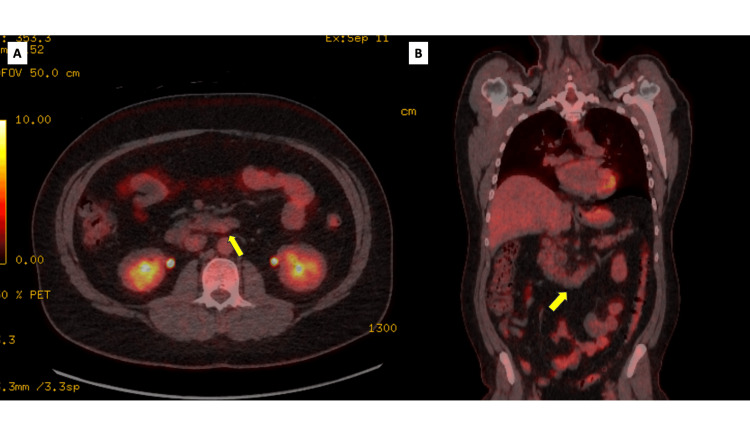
Case 1: FDG-PET imaging of ectopic pancreas. FDG-PET images demonstrating no significant metabolic uptake within the duodenal lesion at the D3/D4 junction (yellow arrow), indicating absence of hypermetabolic activity. (A) Axial view. (B) Coronal view. FDG-PET: fluorodeoxyglucose-positron emission tomography
